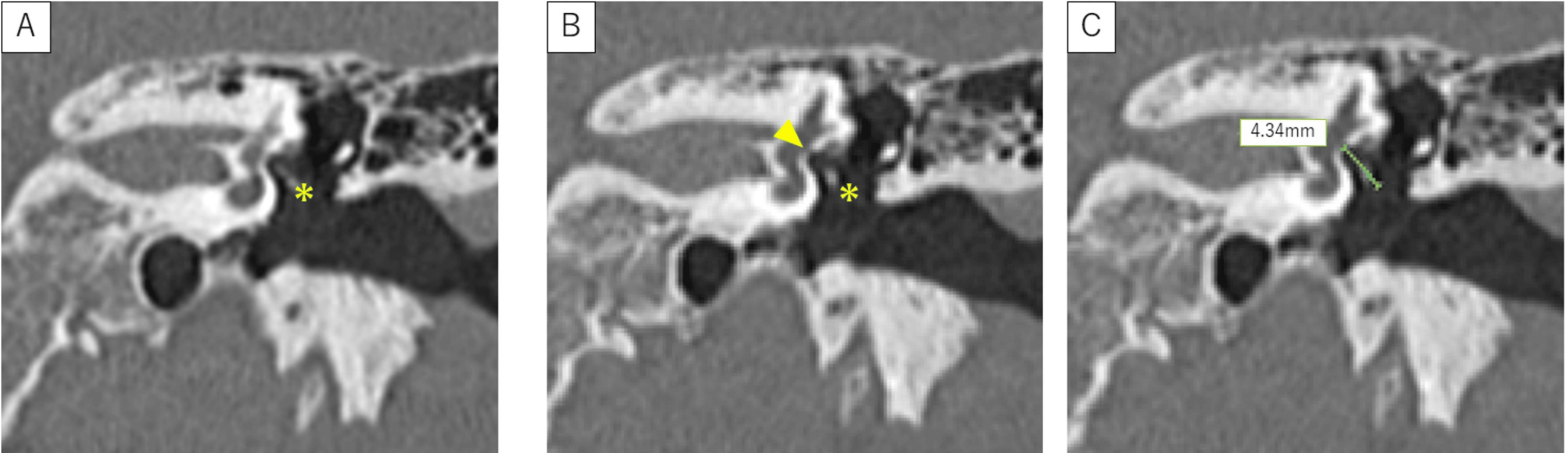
Estimation of the appropriate prosthesis length (eAPL) using thin-slice CT of the left temporal bone (coronal view) A: Identification of the incudostapedial joint on thin-slice CT images obtained under bone window settings. The cursor was fixed at the distal end of the long process of the incus (asterisk). B: Identification of the oval window on an adjacent slice located a few sections away. For coronal measurements, the oval window is defined on the slice in which the stapes footplate was most clearly visualized adjacent to the vestibule and inferior to the facial nerve canal. The straight-line distance from the fixed cursor to the oval window was measured (arrowheads). C: The linear distance between the cursor position in (A) and the oval window represents the estimated appropriate prosthesis length (eAPL).
